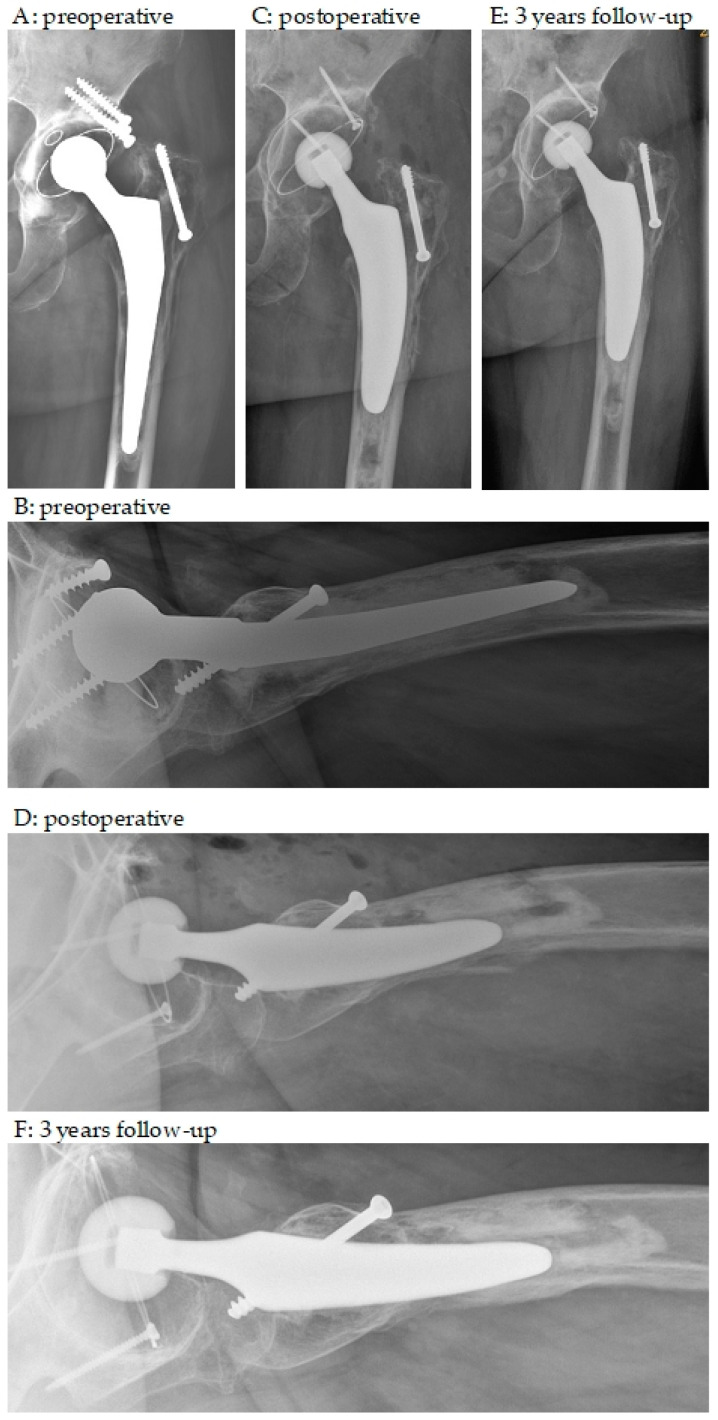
Anteroposterior and axial X-rays. (A,B) preoperative; (C,D) postoperative; (E,F) 3 year follow-up. A 79-year-old woman with aseptic loosening of a cemented stem and cup and eccentric PE wear; 27 years after primary THA; cup and stem revision with pressfit monobloc cup, additional screw fixation and cementless short stem; intraoperative posterior femoral perforation during cement removal with weak bone; fearing further perforation without osteotomy distal cement plug was left in place; last follow-up with healed bone and fixed short stem in place.
